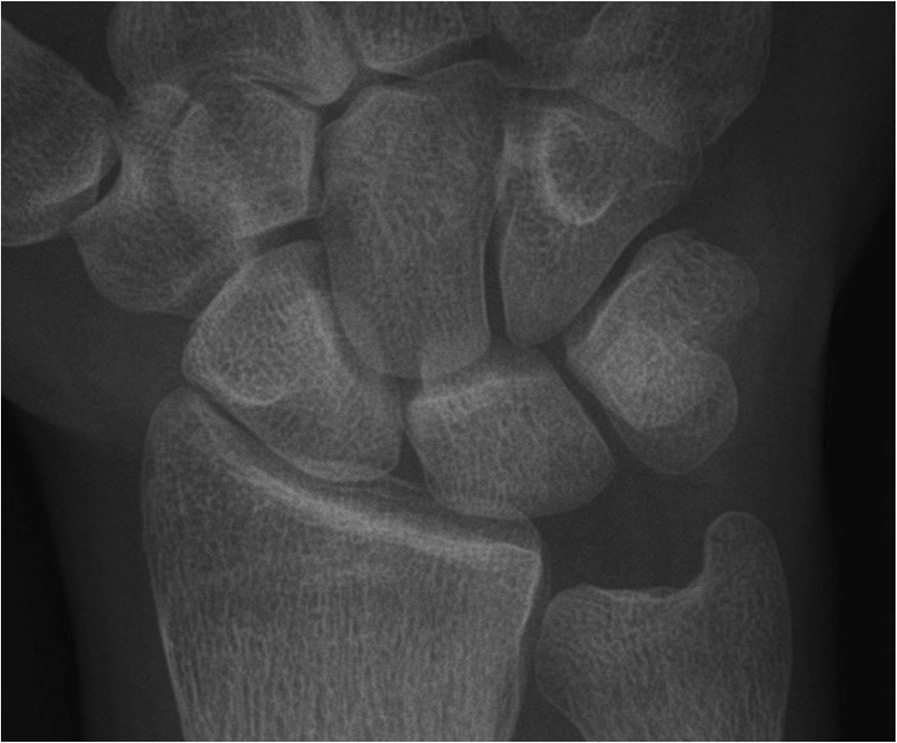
Pre-operative pa x-rays of the right wrist of a 31-year-old male patient who had undergone RSO
